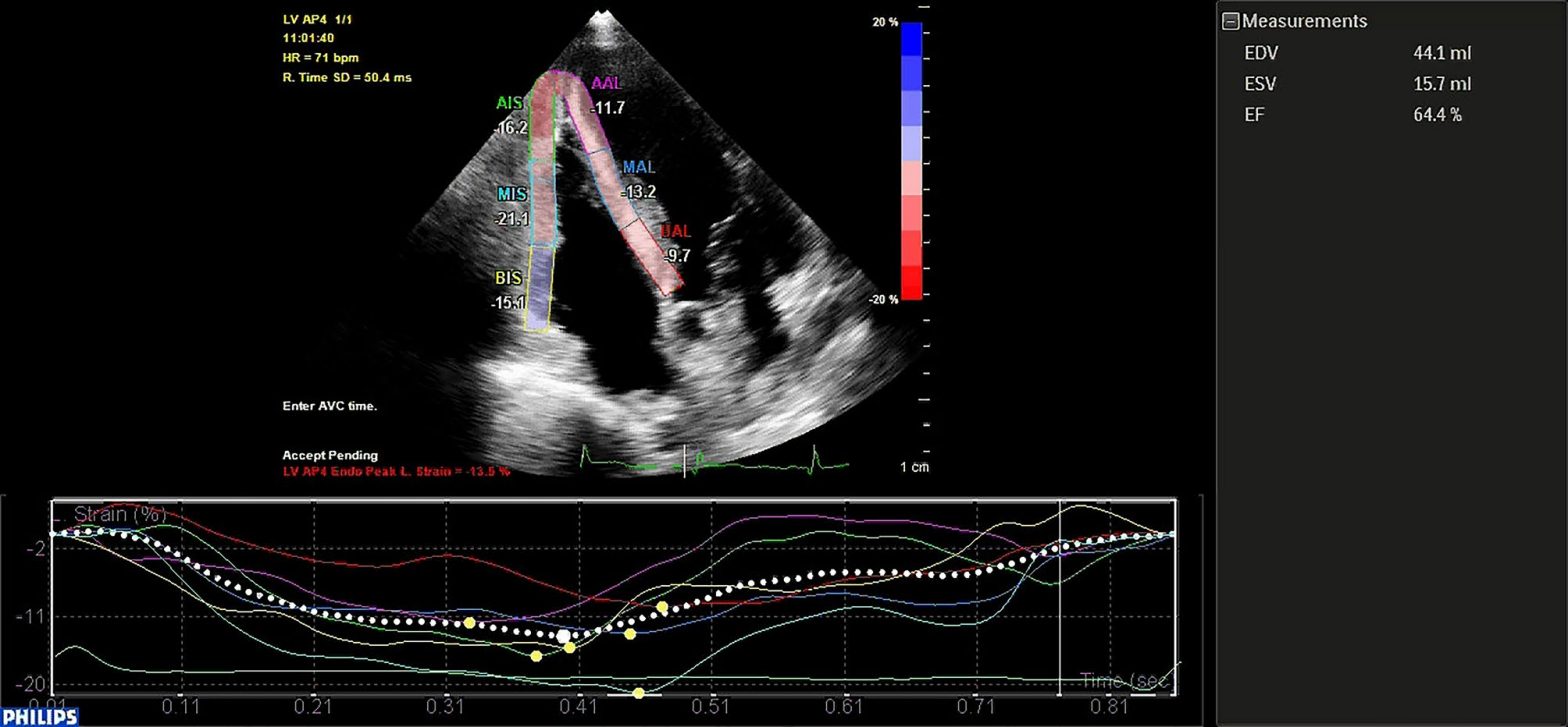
Speckle-tracking echocardiography analysis in an OSAS patient: the right ventricle is divided in 6 segments: 3 on the free wall and 3 in the septum.
